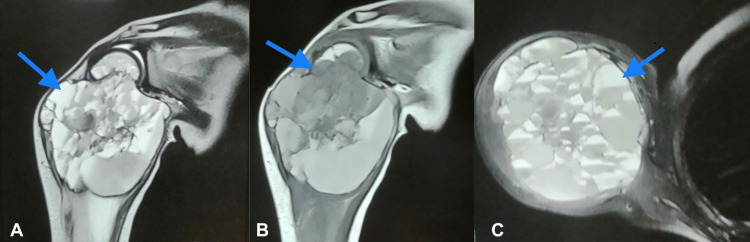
MRI T1/T2-weighted of right shoulder showing cystic lesion arising from proximal shaft humerus in coronal section (A, B) and axial section (C).
